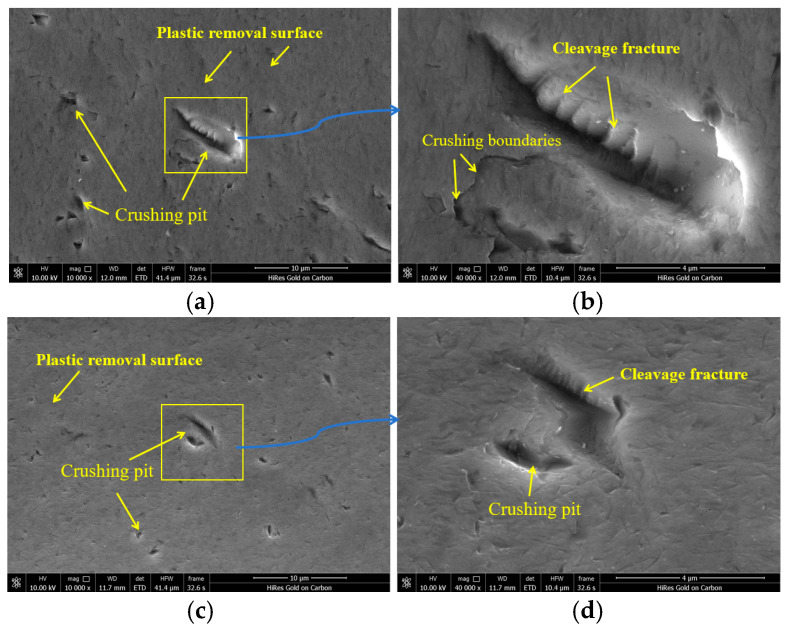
Typical surface morphology of the monocrystal silicon after polishing: (a) overall morphology without ultrasonic assistance; (b) local morphology without ultrasonic assistance; (c) overall morphology with ultrasonic assistance; (d) local morphology with ultrasonic assistance.
